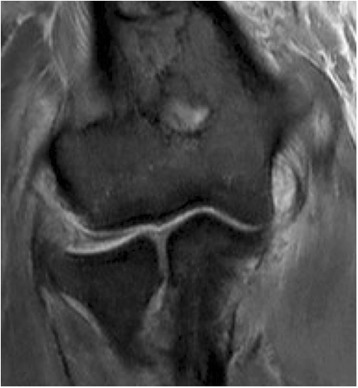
Coronal view in T2-weighted MRI after simple elbow dislocation: The MCL was rated to be intact (1×), partially torn (1×) and completely torn (2×)
